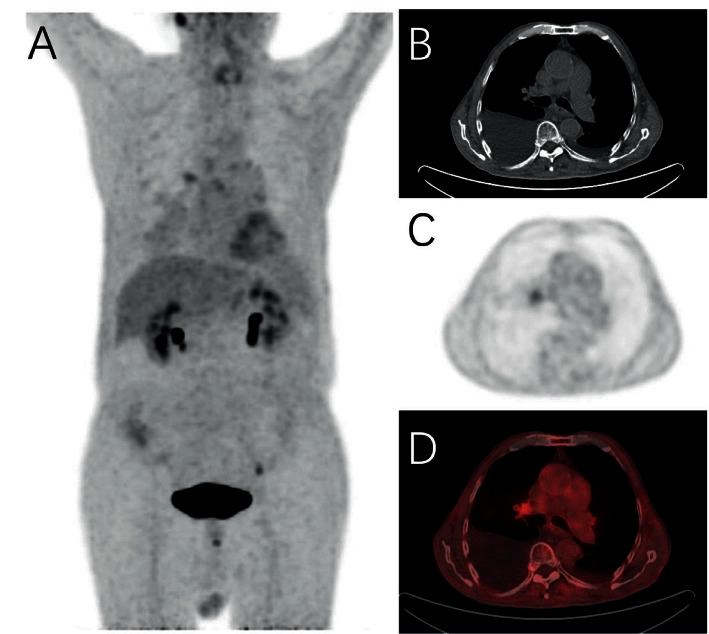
A 74-year-old man presented with chest tightness and was diagnosed with TBP by thoracoscopic biopsy. (b) Bilateral pleural effusion. (a, c, d) Normal 18F-FDG uptake of both pleurae.
